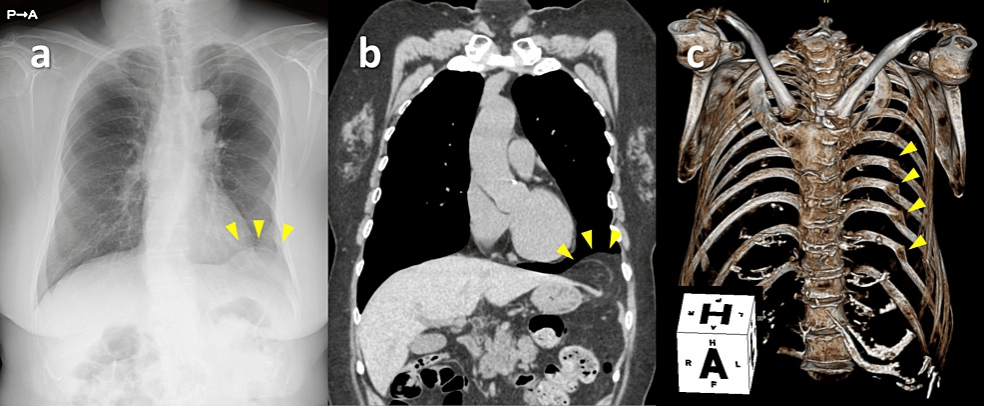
Imaging examinations. a) Chest radiography revealed a sharply demarcated soft tissue shadow on the left diaphragm. b) Computed tomography identified a diaphragmatic defect of approximately 2 cm and a protrusion of the greater omentum into the thoracic cavity. c) Healed fractures of the left eighth to 11th ribs were identified.
